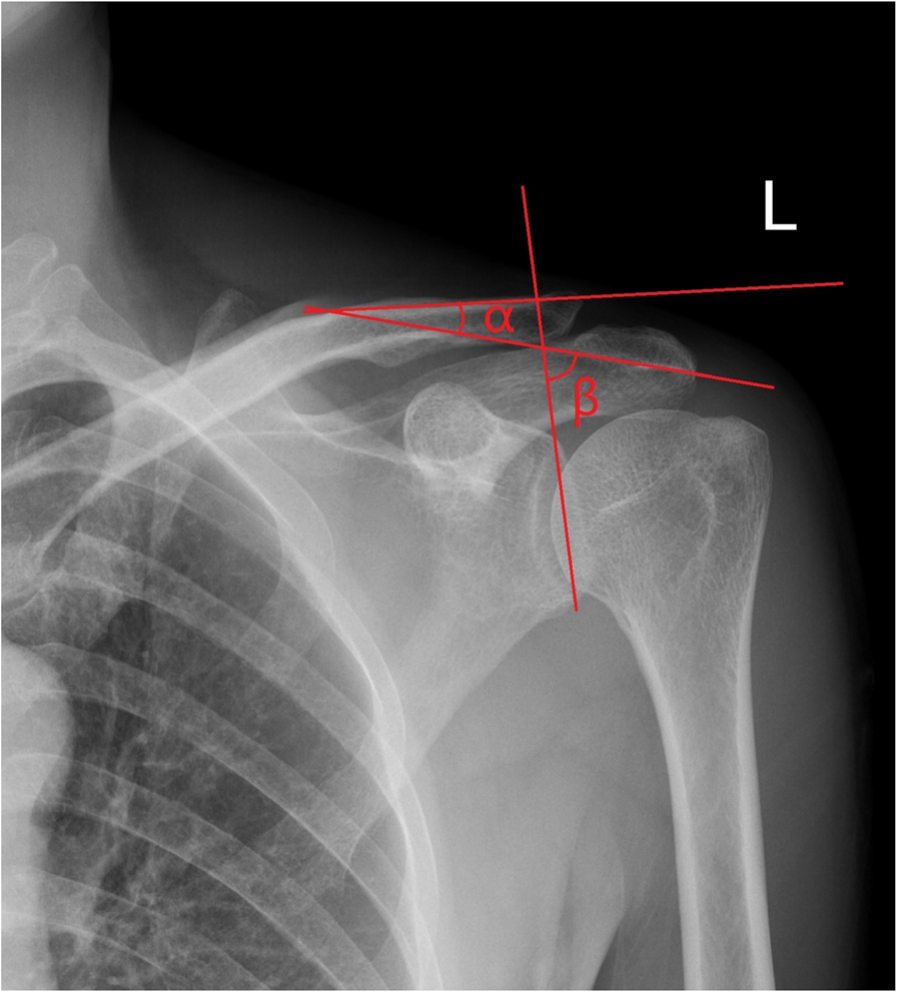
Calculate the distal clavicle–acromion coronal angle (α) and acromion coronal angle (β) on a standardized true anteroposterior radiograph
